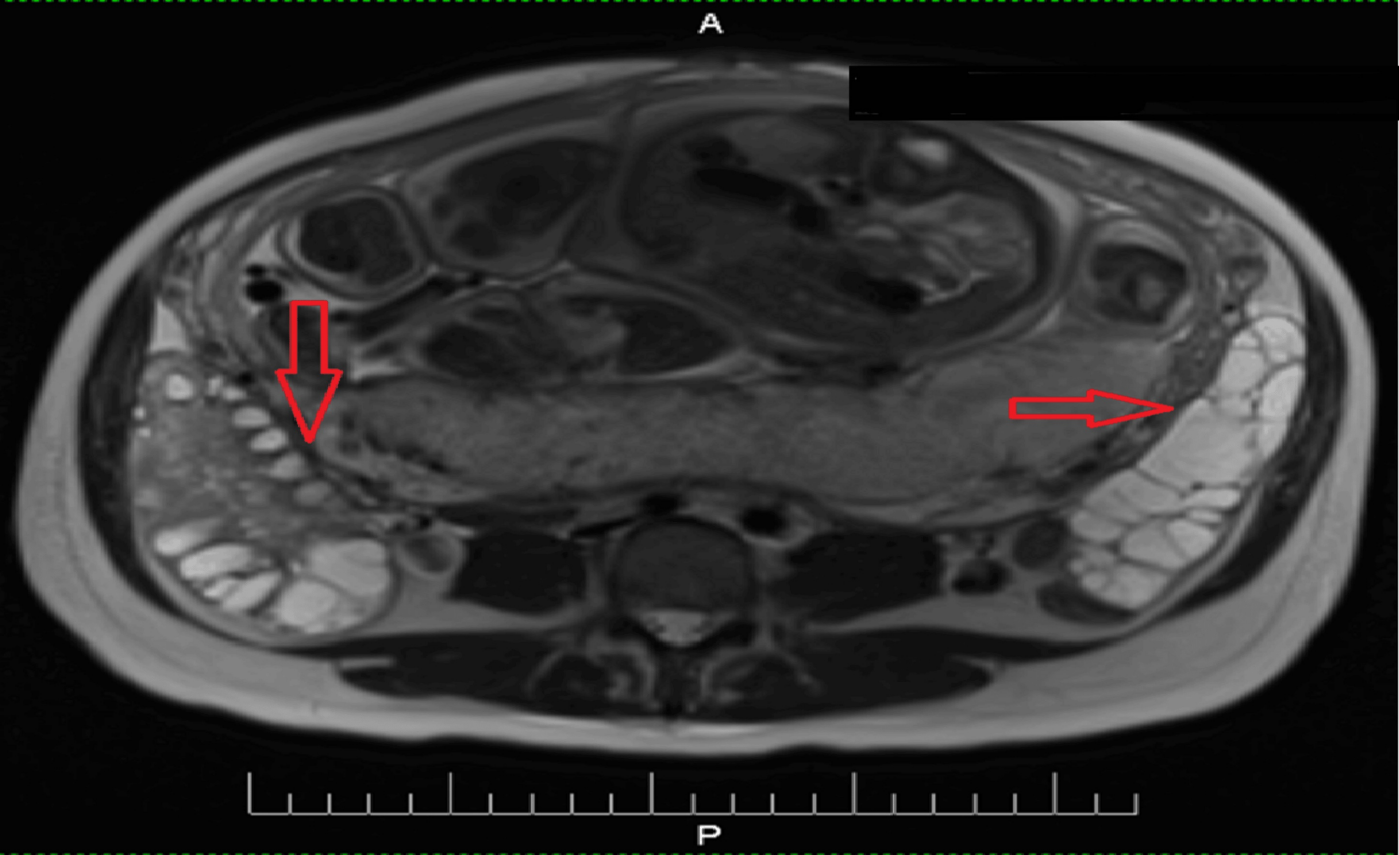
MRI image of enlarged ovaries A: anterior; P: posterior
